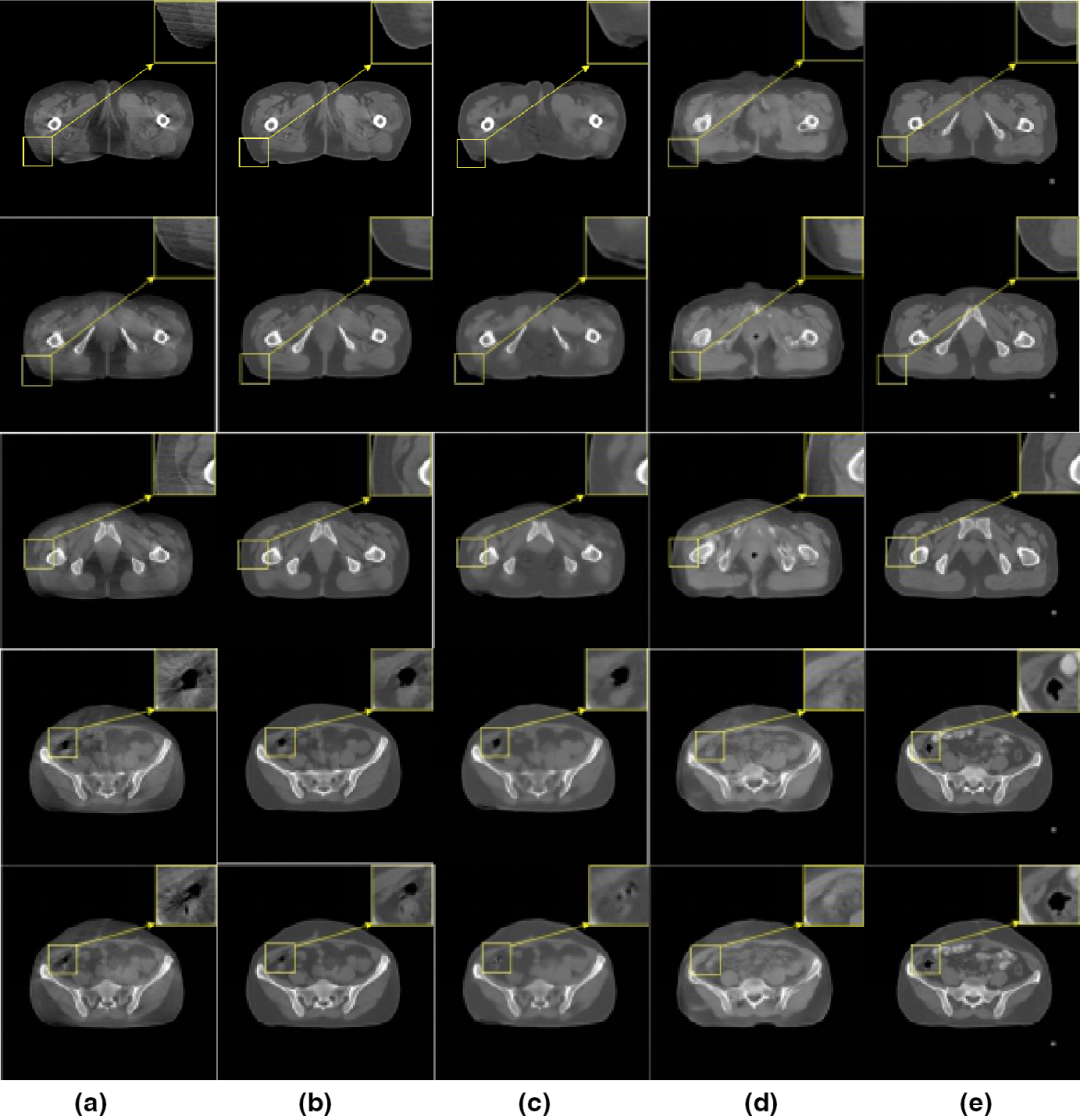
Artifact removal results obtained with the pelvis data. (a) CBCT, (b) proposed correction, (c) method in 21, (d) method in 32, and (e) RCT. Display window [−360, 628].
